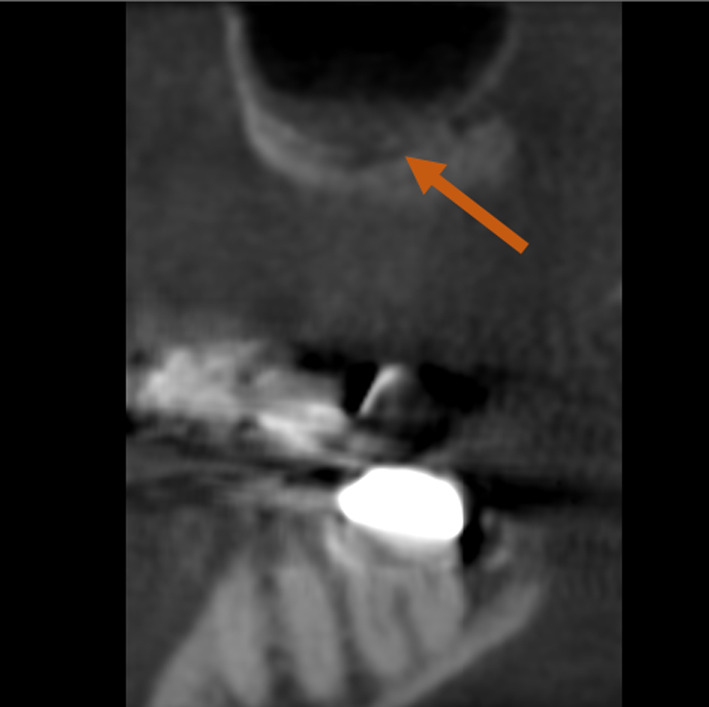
CBCT images with AAA canal of the right maxillary sinus, sagittal slice
